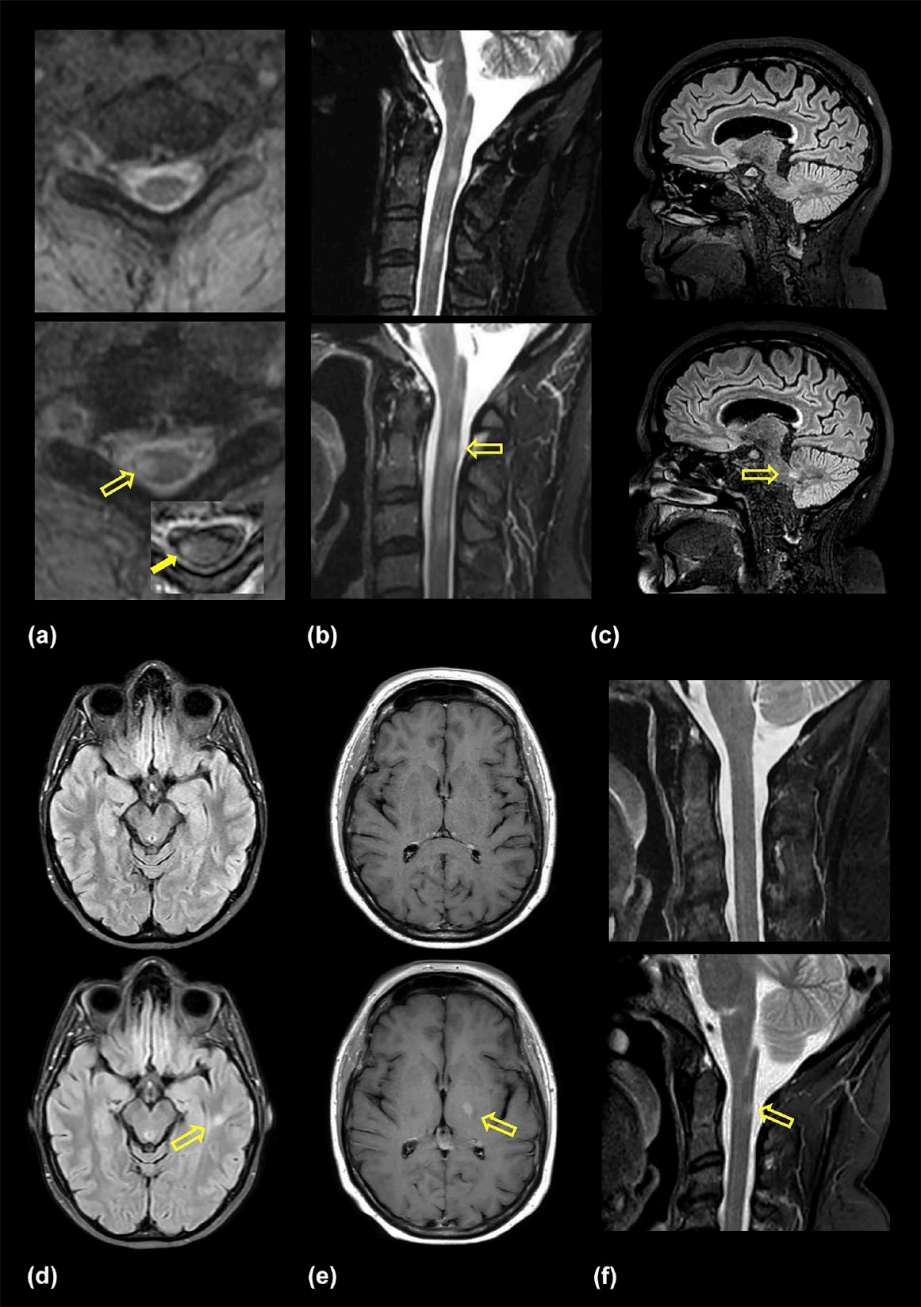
Magnetic resonance imaging data from the six people with MS included in this report with A-F providing MRI evidence of disease advancement. (a) Axial T2 MRI of the cervical spinal cord before (upper panel) and after relapse demonstrating a new T2-weighted hyperintense lesion (lower panel; open yellow arrow) with gadolinium enhancement (inset, lower panel image (solid yellow arrow)). (b) Sagittal short tau inversion recovery (STIR) sequence of the cervical spinal cord demonstrating enlargement of the prior intramedullary lesion at C2 (lower panel; open yellow arrow). Gadolinium was not administered during this study. Note the increase in the T2-weighted signal and caliber of the spinal cord when comparing the upper and lower panels. (c) Sagittal 3-dimensional FLAIR image revealing the presence of a new cerebellar peduncle T2-weighted focus (lower panel; open yellow arrow) when compared to prior MRI data (upper panel). (d) Axial FLAIR images of the brain revealing a new left temporal white matter lesion (lower panel; open yellow arrow). (e) Postgadolinium T1-weighted images highlighting the presence of a deep gray matter (basal ganglia/internal capsule) contrast enhancing lesion (lower panel; open yellow arrow) when compared to prior data (upper panel). (f) Sagittal STIR sequence of the cervical spine demonstrating a new intramedullary T2-weighted hyperintense focus at C2 (lower panel; open yellow arrow) when compared to the prior study (upper panel). FLAIR, fluid attenuated inversion recovery; MS, multiple sclerosis; STIR, short tau inversion recovery.
